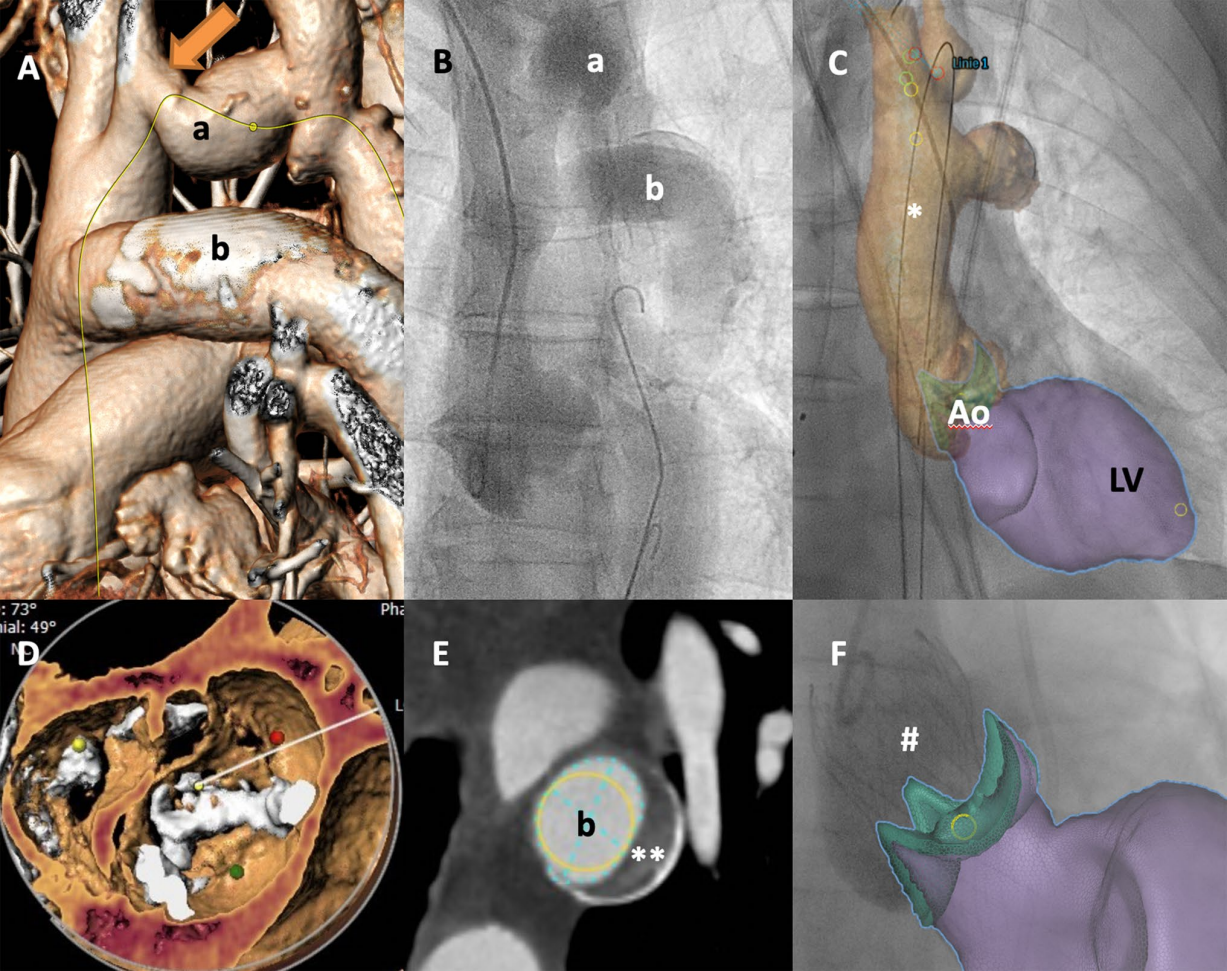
A CT-reconstruction of “double” aortic arch: elongated, stenotic native aortic arch (a) and aorto-aortic bypass (b), arrow indicating smallest vessel diameter of the coarctation. B Fluoroscopy (via radial pigtail-catheter, tip in non-coronary sinus): native aortic arch (a) and aorto-aortic bypass (b). C Fusion imaging (LV, left ventricle; Ao, aortic valve), stiff wire (*) guided by real-time fusion imaging through native stenotic aortic arch (color markers indicating stenotic segment). D CT-reconstruction of native aortic valve stenosis (n. b. calcified fusion of left and right coronary cusp/bicuspid aortic valve Typ I LR). E CT-reconstruction of aorto-aortic bypass (b) with appositional thrombus (). F Fusion imaging: result aortography showing self-expanding TAVR-prosthesis (#) in situ
